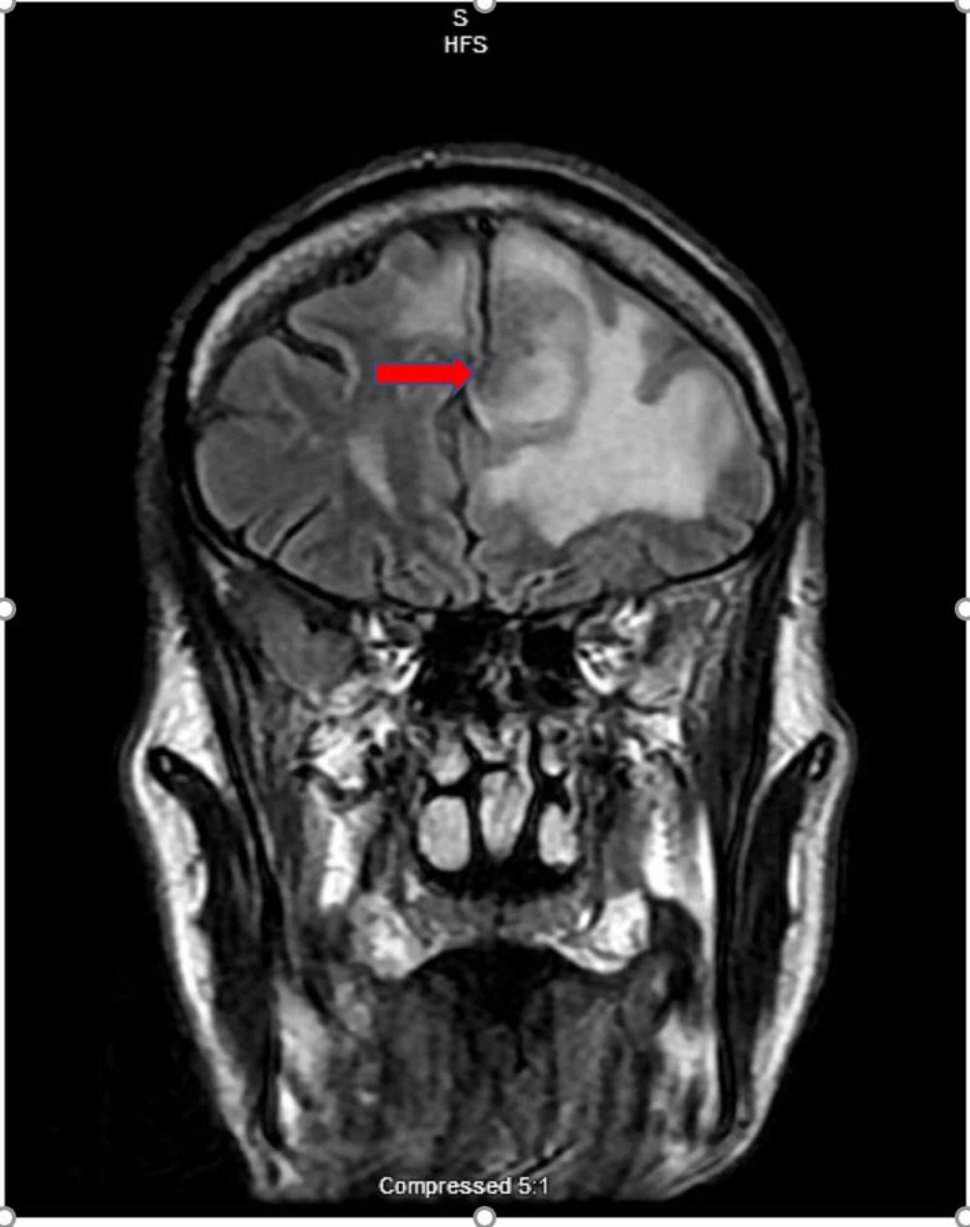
MRI brain, coronal flair section. Red arrow showing frontal lesion with surrounding edema.
